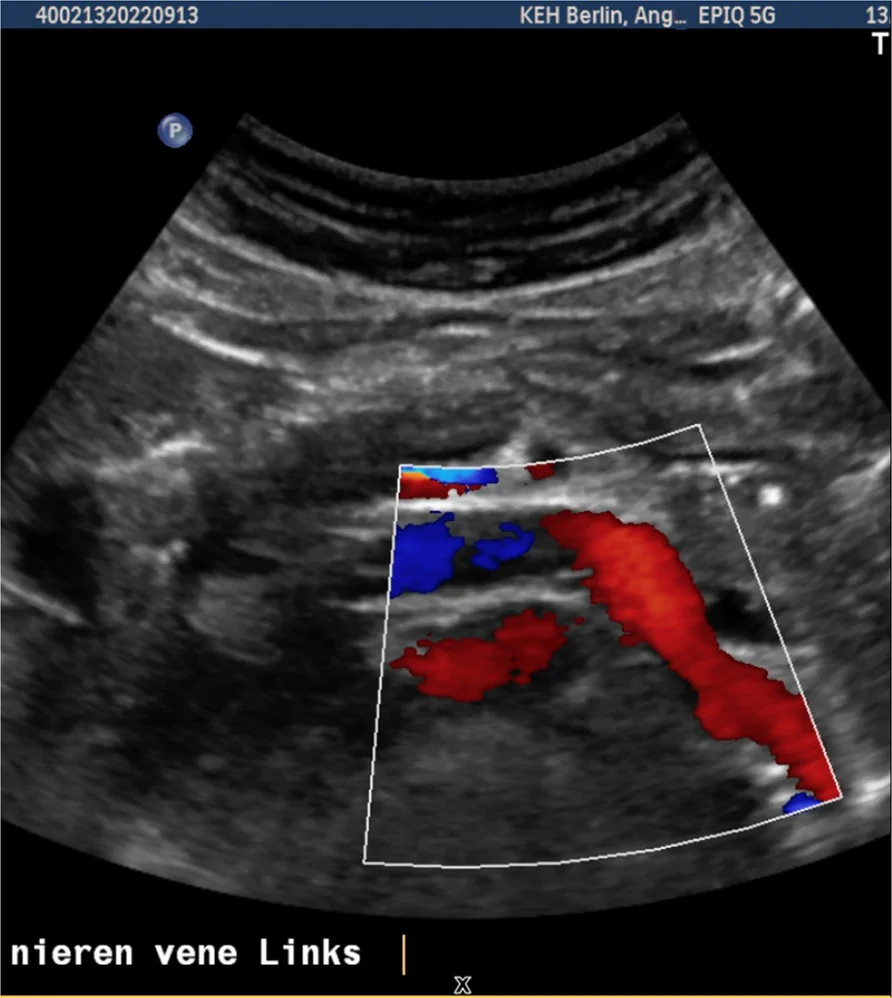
Ultrasound 2 years following device placement demonstrating full patency
